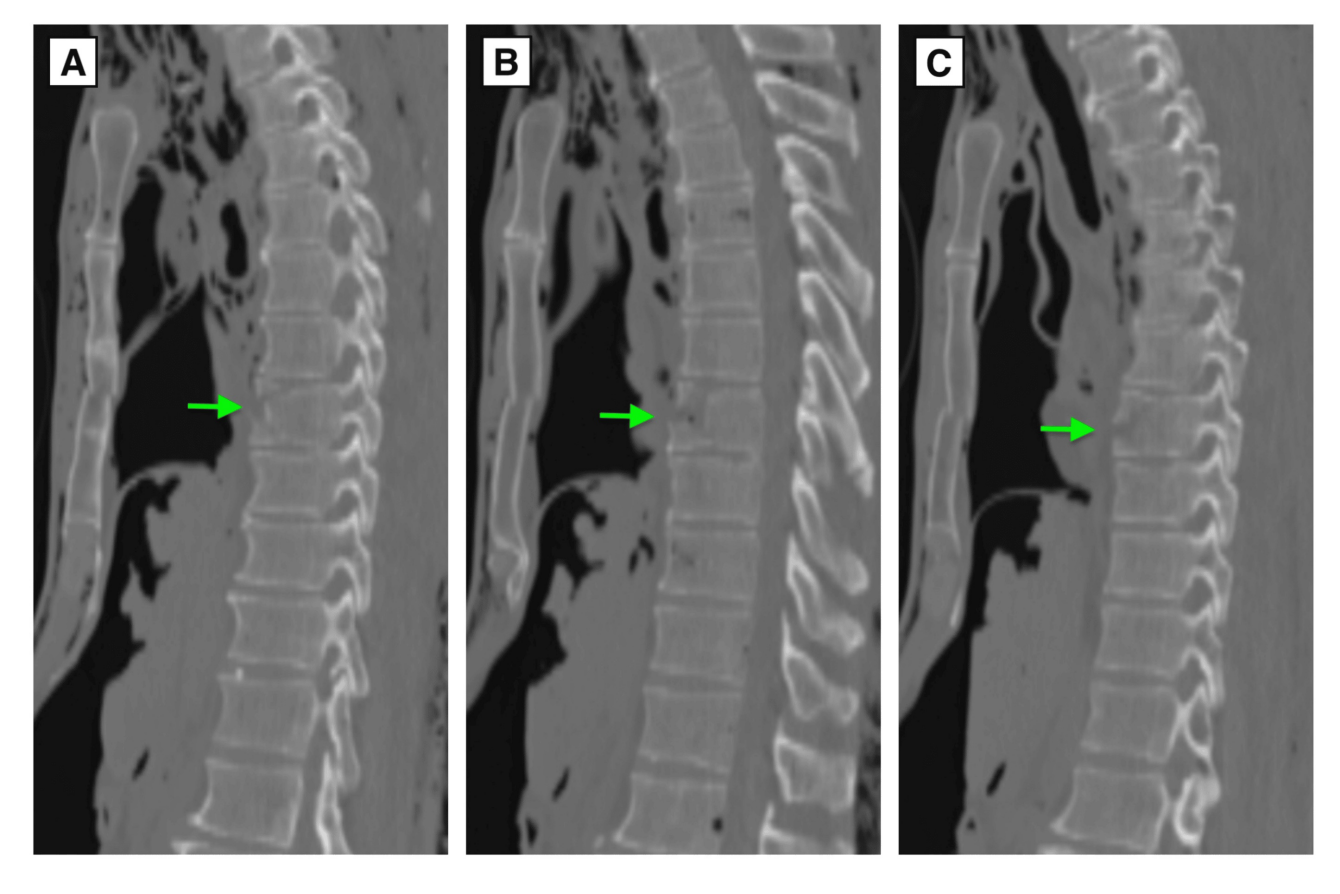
Sagittal CT images of the sixth thoracic vertebra (Case 2). (A) Right pedicle level. The arrow indicates the fracture site. (B) Midline. The arrow indicates the fracture site. (C) Left pedicle level. The arrow indicates the fracture site.
